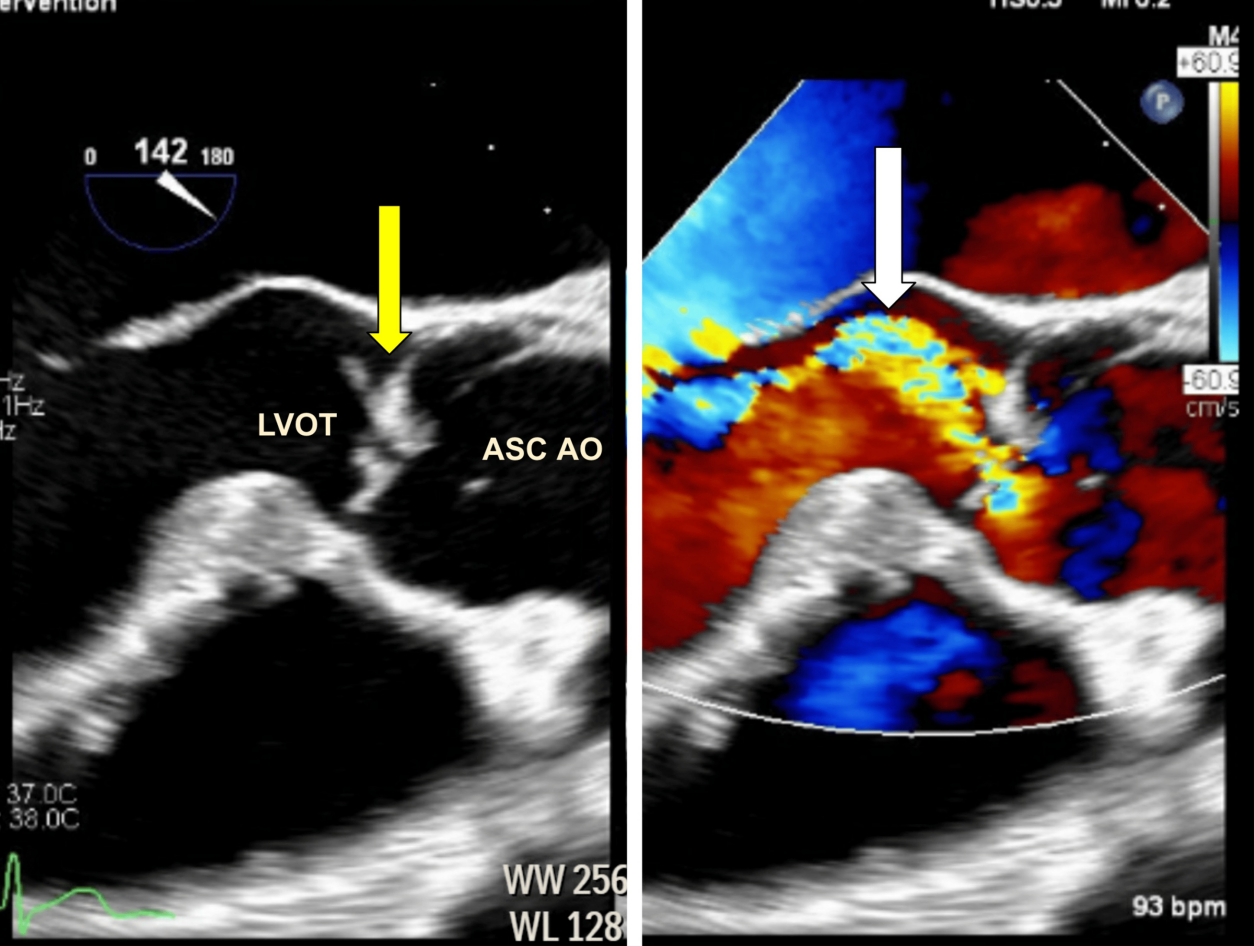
Transesophageal echocardiogram (left) depicting an echogenic area involving the right and left coronary cusp, consistent with endocarditis (yellow arrow). Color flow study (right) revealing moderate-to-severe aortic regurgitation secondary to turbulent flow (white arrow). LVOT: left ventricular outflow tract; ASC AO: ascending aorta.
